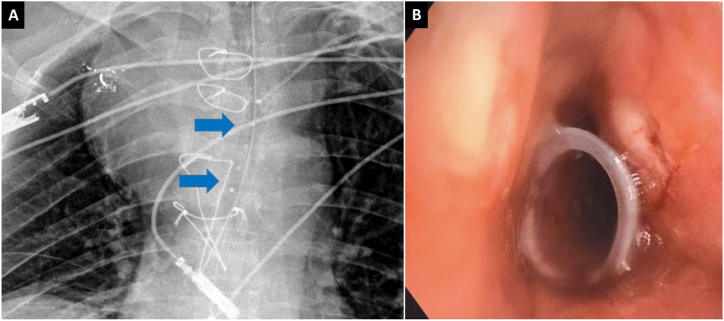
A: Chest X-ray after silicone stent placement in the distal trachea, radiopaque stent buttons (blue arrows) are observed. B: Bronchoscopy: the proximal end of the silicone stent is observed in the middle trachea.
